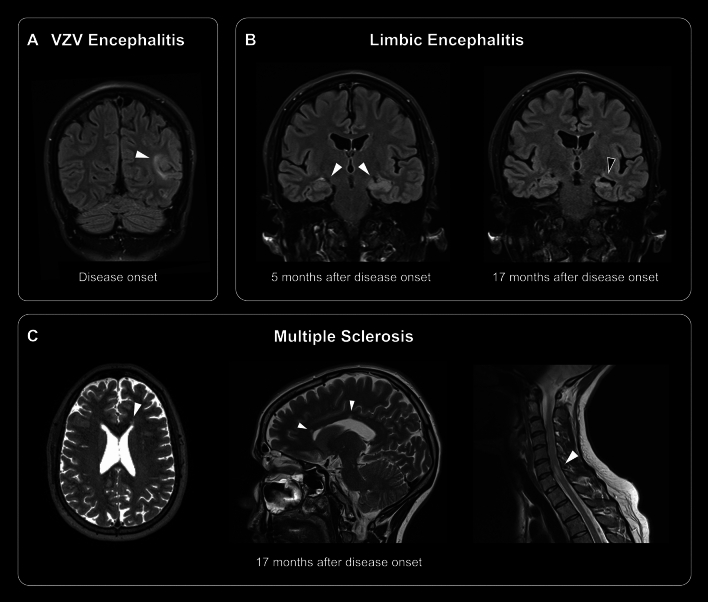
Cerebral and spinal MRI findings. At onset of VZV encephalitis, a hyperintense lesion under the left angular gyrus was present (panel A). Five months later, limbic encephalitis manifested with bilateral (left > right) hippocampal FLAIR hyperintensity, which reverted to left hippocampal atrophy under immunomodulating treatment (panel B). Seventeen months after onset of VZV encephalitis and twelve months after onset of limbic AIE, new periventricular, callosal, and spinal T2 hyperintense lesions supported the additional diagnosis of multiple sclerosis (panel C). White arrows indicate FLAIR hyperintense lesions, black arrow indicates atrophy. *AIE* autoimmune encephalitis; *MRI* magnetic resonance imaging; *FLAIR* fluid attenuated inversion recovery; *VZV* varicella zoster virus
